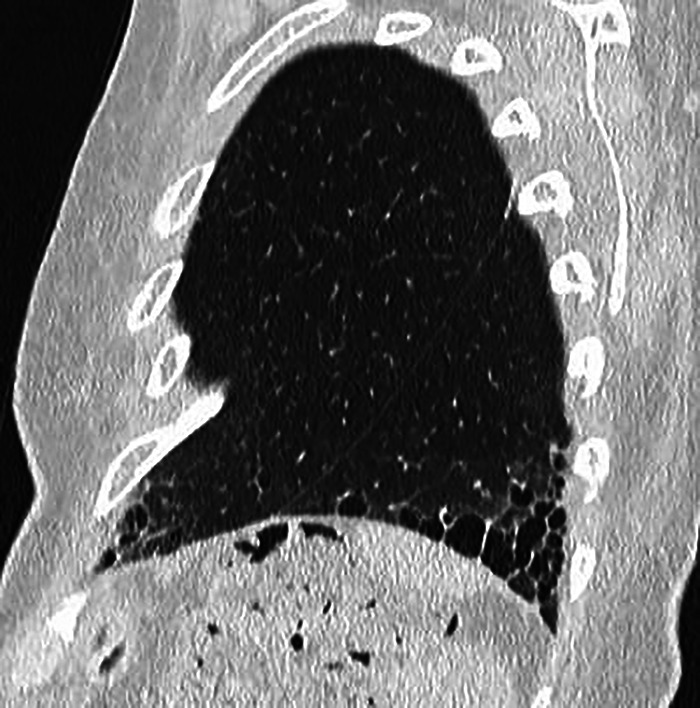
Sagittal follow-up CT image of lungs showing exuberant and macrocystic honeycomb at the periphery of the pulmonary bases, increasing compared to previous exam.
